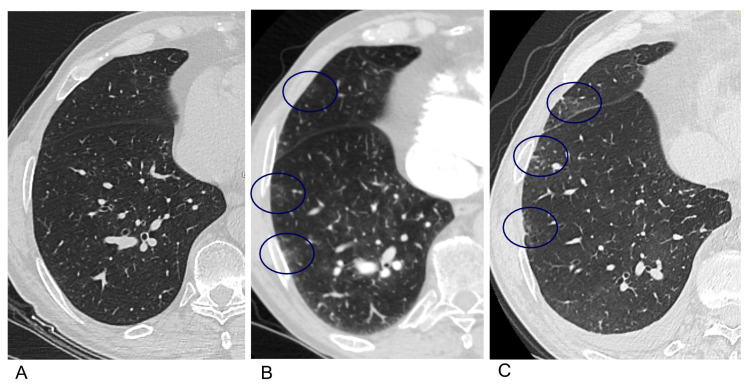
Evolution of chest computed tomography pulmonary angiography (CTPA) features before and after COVID-19 pneumonia in a patient with pulmonary hypertension. (A) Chest CTPA image (lung window) before COVID-19 pneumonia, no changes in the lung parenchyma. (B) Two-month follow-up CTPA after COVID-19 pneumonia: subtle ground-glass opacities in the periphery of the parenchyma (circles). (C) Follow-up CTPA after 2 years: typical fibrotic lung changes after COVID-19 (subpleural reticulations with subtle ground glass in the basal lung parts (circles)).
